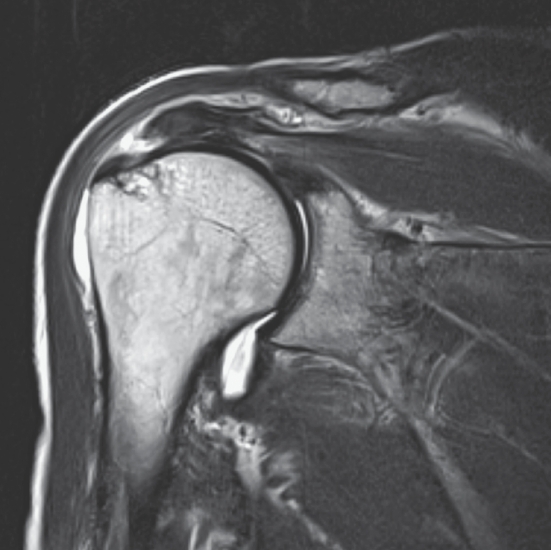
Case presentation. Magnetic resonance image (T2WI) of a shoulder showing rotator cuff tear and glenohumeral effusion.
